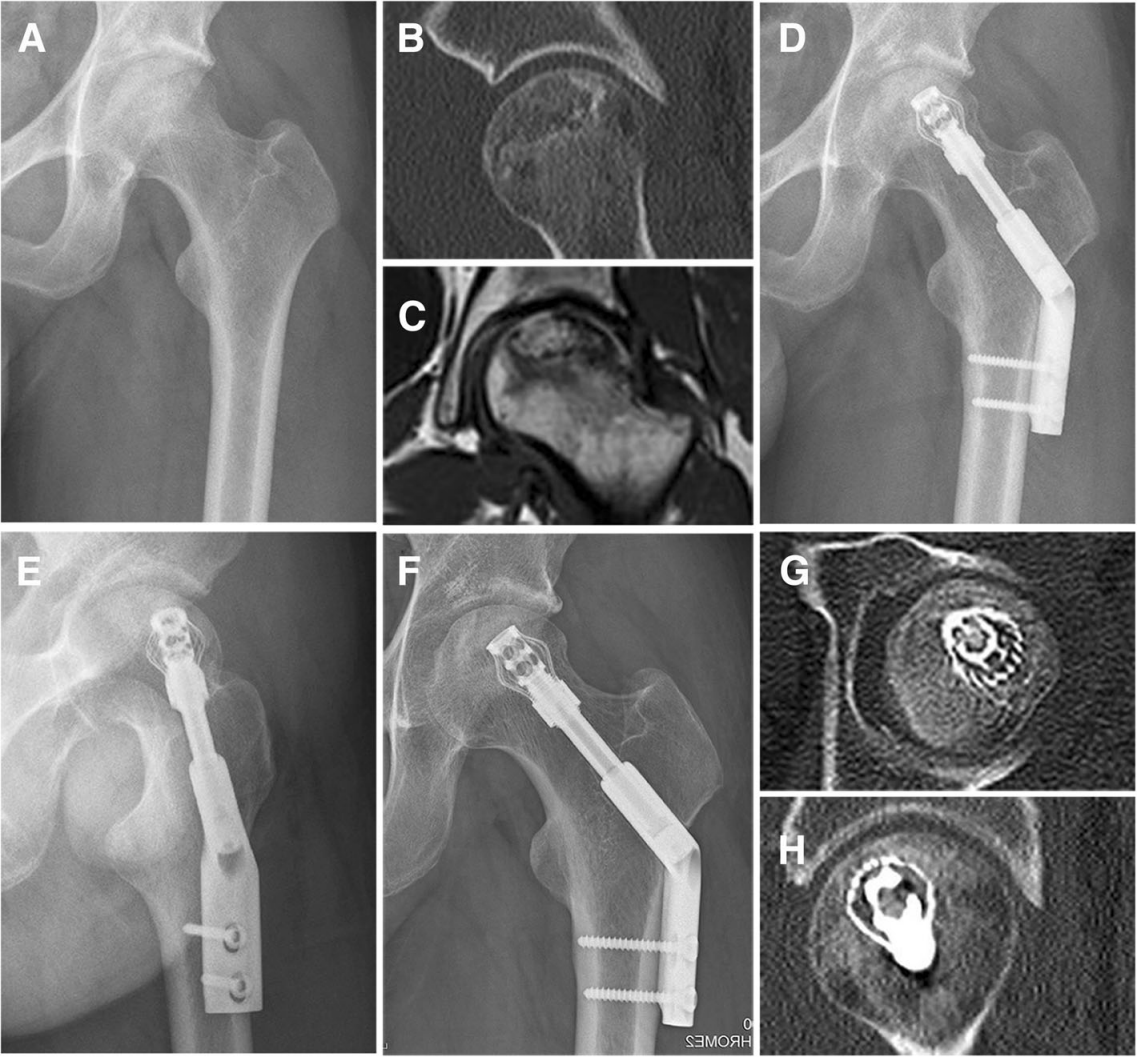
Representative radiographic images from both preoperative and postoperative taken at immediately after the lantern-shaped screw implantation and 36 months. a-c Preoperative X-ray, CT and sagittal T2-weighted magnetic resonance image showing ARCO stage III ONFH in a man aged 29 years. d and e X-ray anteroposterior view on the day of surgery. f-h X-ray and CT scans at 36 months after surgery showing union
